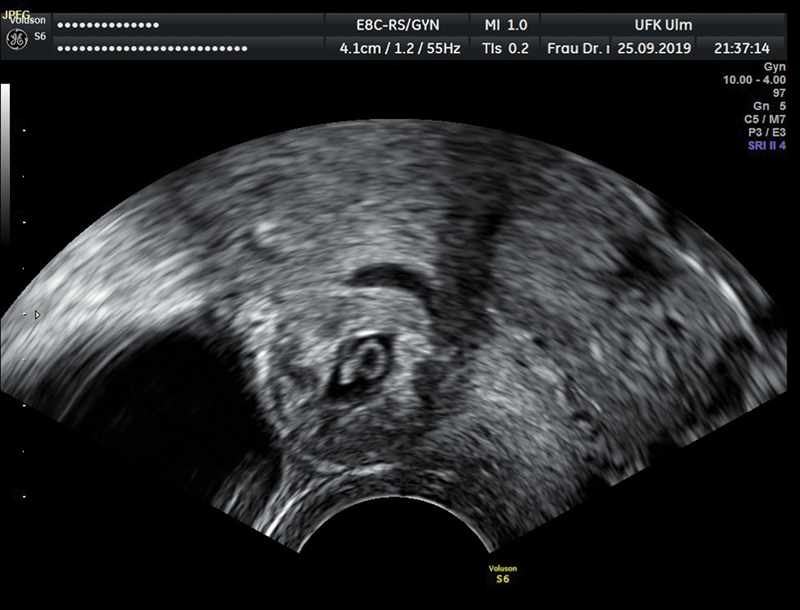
Ultrasound picture of a scar pregnancy.
